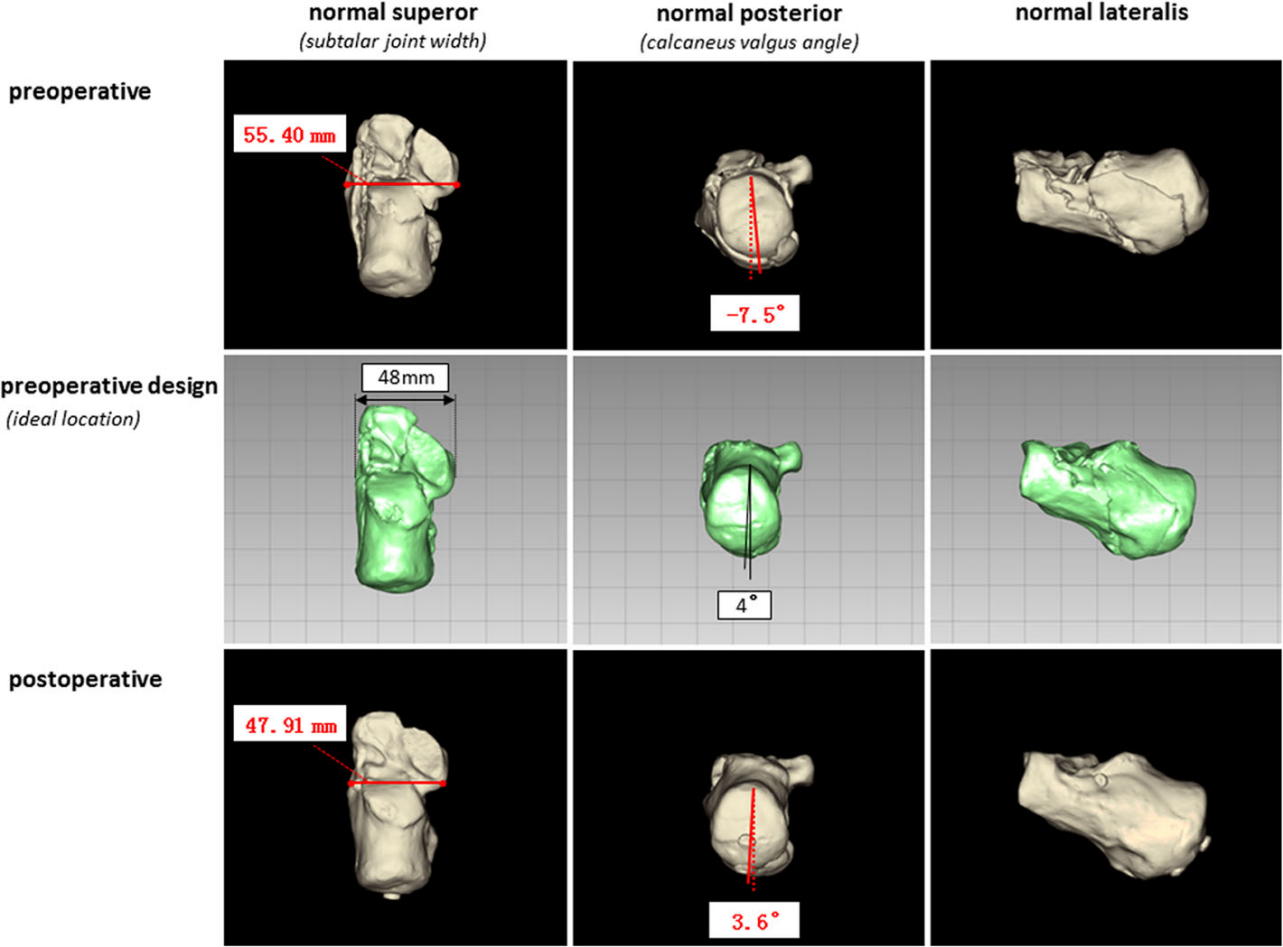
3D Reconstructed model generated based on the preoperative plan and pre- and postoperative actual measurements from CT data. We measured subtalar joint width at the position of the sustentaculum from the normal superior perspective, and the calcaneus valgus angle from the normal posterior perspective
